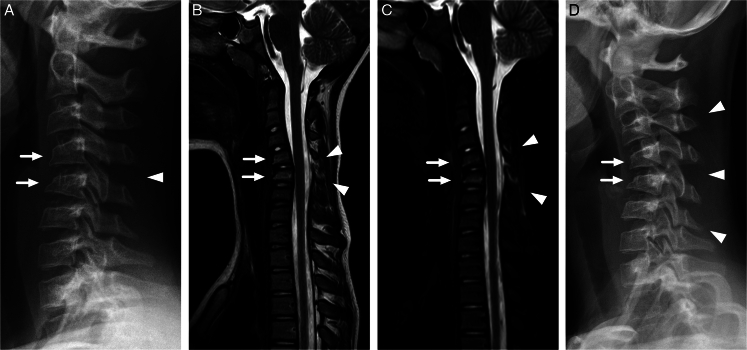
Posterior ligamentous complex injury and vertebral compression fractures in a 10-year-old girl after a fall. A, Primary lateral plain radiograph from an outside hospital with vertebral compression fractures (arrows), interspinous distance widening (arrowhead), and abnormal kyphosis. Sagittal T2-weighted series (B) and Sagittal short tau inversion recovery series from emergency magnetic resonance imaging show vertebral compression fractures (arrows) and posterior ligamentous complex injury with abnormal kyphosis (C). A facet joint capsule injury was also present. D, Follow-up lateral plain radiograph after a 1-week attempt of conservative treatment with a rigid cervical collar demonstrates the progression of the traumatic kyphotic malalignment. The surgical fixation was performed.
